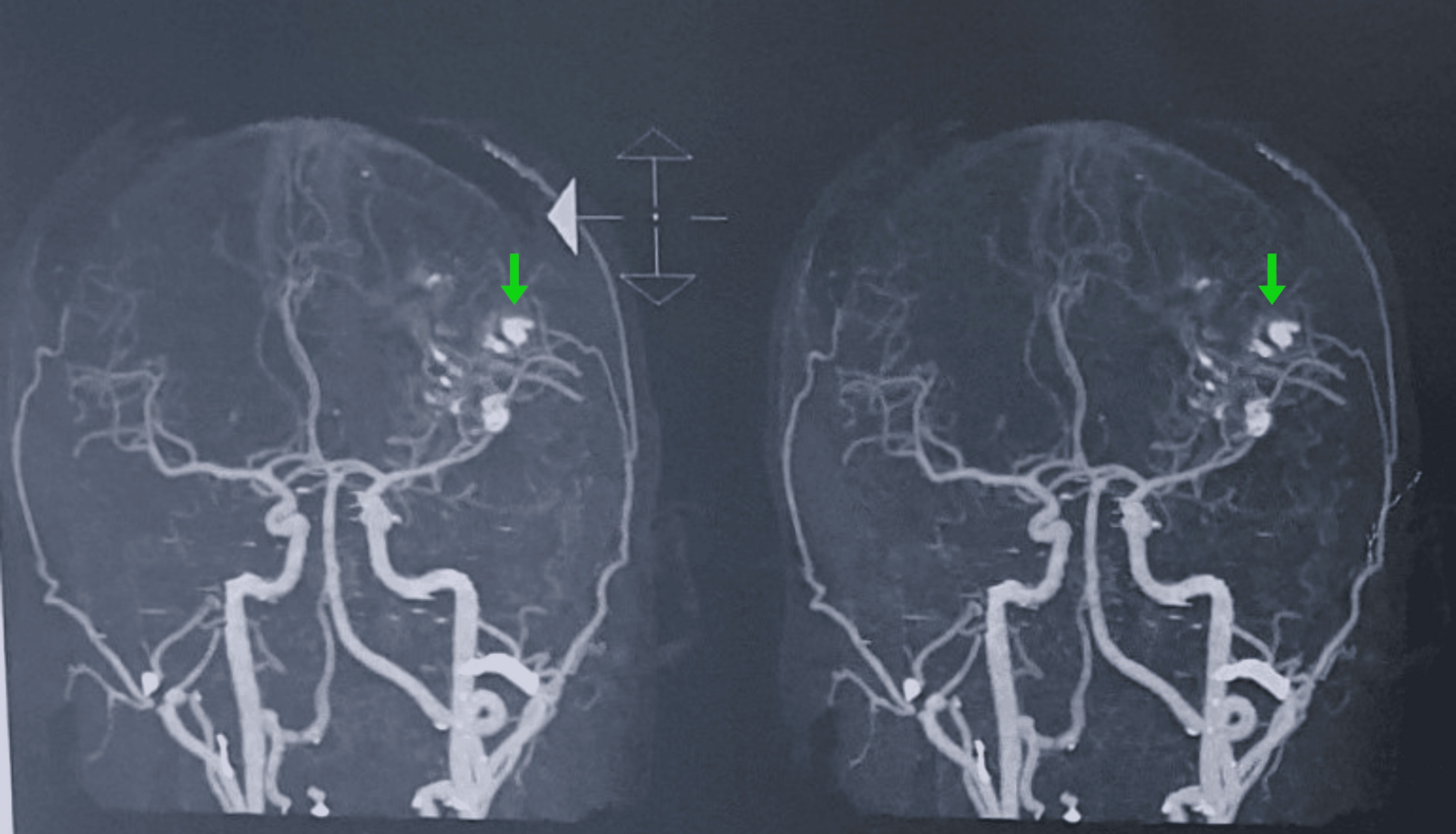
Coronal CT angiography Showing sequential sections of cerebral vessels with gyriform cortico-subcortical (“tram-track”) calcifications in the left hemisphere (green arrows).
